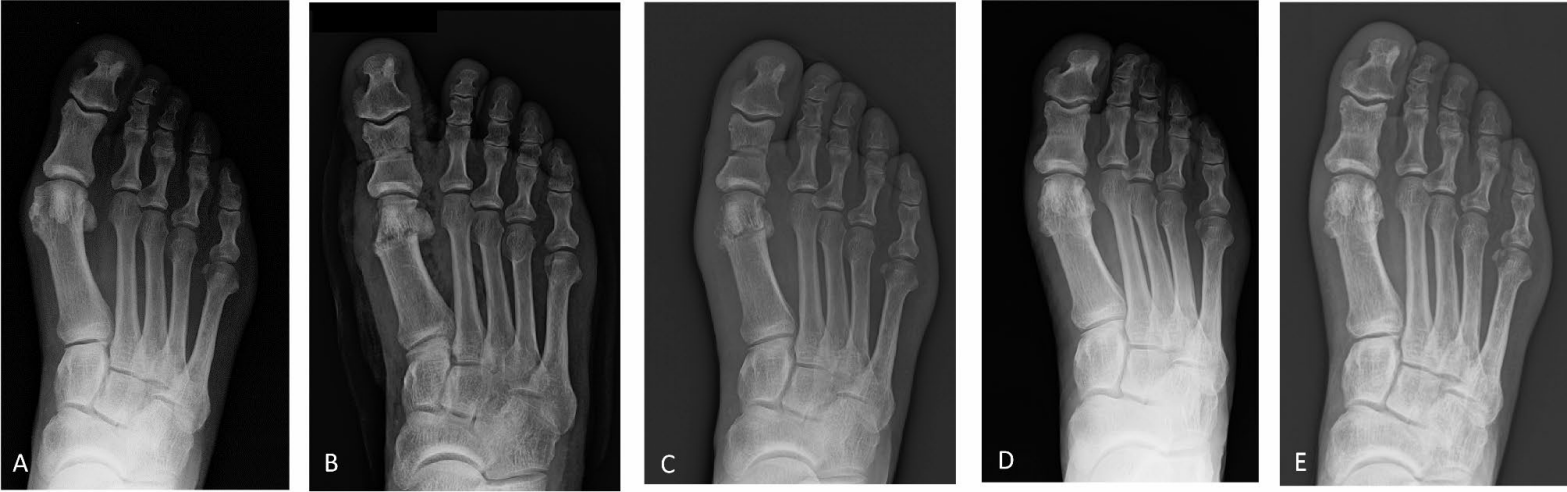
Case 1: A 69-year-old man having undergone the Reverdin-Isham and Akin osteotomies for moderate HV correction of his right foot. Weightbearing radiographic images of anteroposterior view at (A) preoperative period, (B) 1-month follow-up, (C) 3-month follow-up, (D) 12-month follow-up, and (E) last follow-up at 60 months after surgery, showing bone callus consolidation and remodeling, a stable and long-lasting radiographic correction
